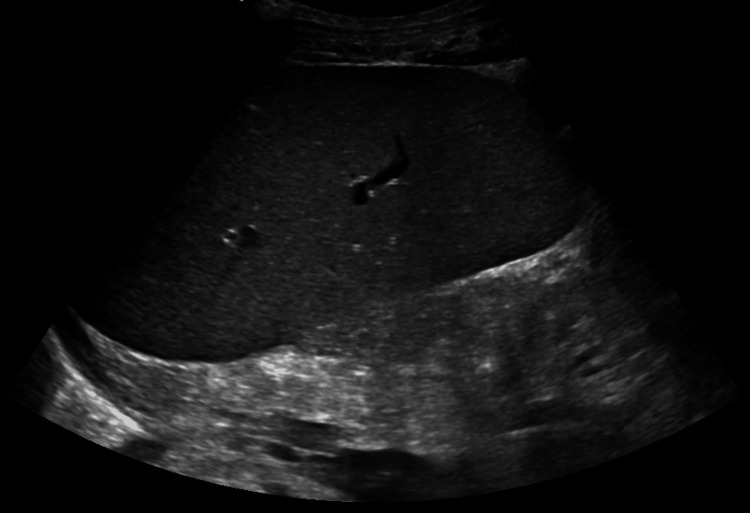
Abdominal ultrasound demonstrating splenomegaly (15.2 x 7.2 x 7.5 cm).
